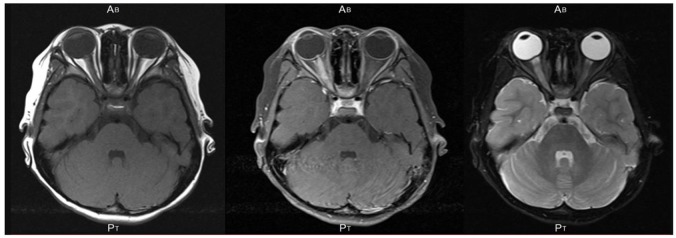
An axial section of magnetic resonance images of the brain and orbits in the patient showing hyperintensity of the right optic nerve on short TI inversion recovery (STIR) sequence (Left), T1-weighted image with gadolinium enhancement (Middle), and T2-weighted image (Right). No cerebral lesion was detected. An axial section of MRI of the brain and orbits in the patient showing hyperintensity of the right optic nerve on short TI inversion recovery (STIR) sequence and no cerebral lesion.
